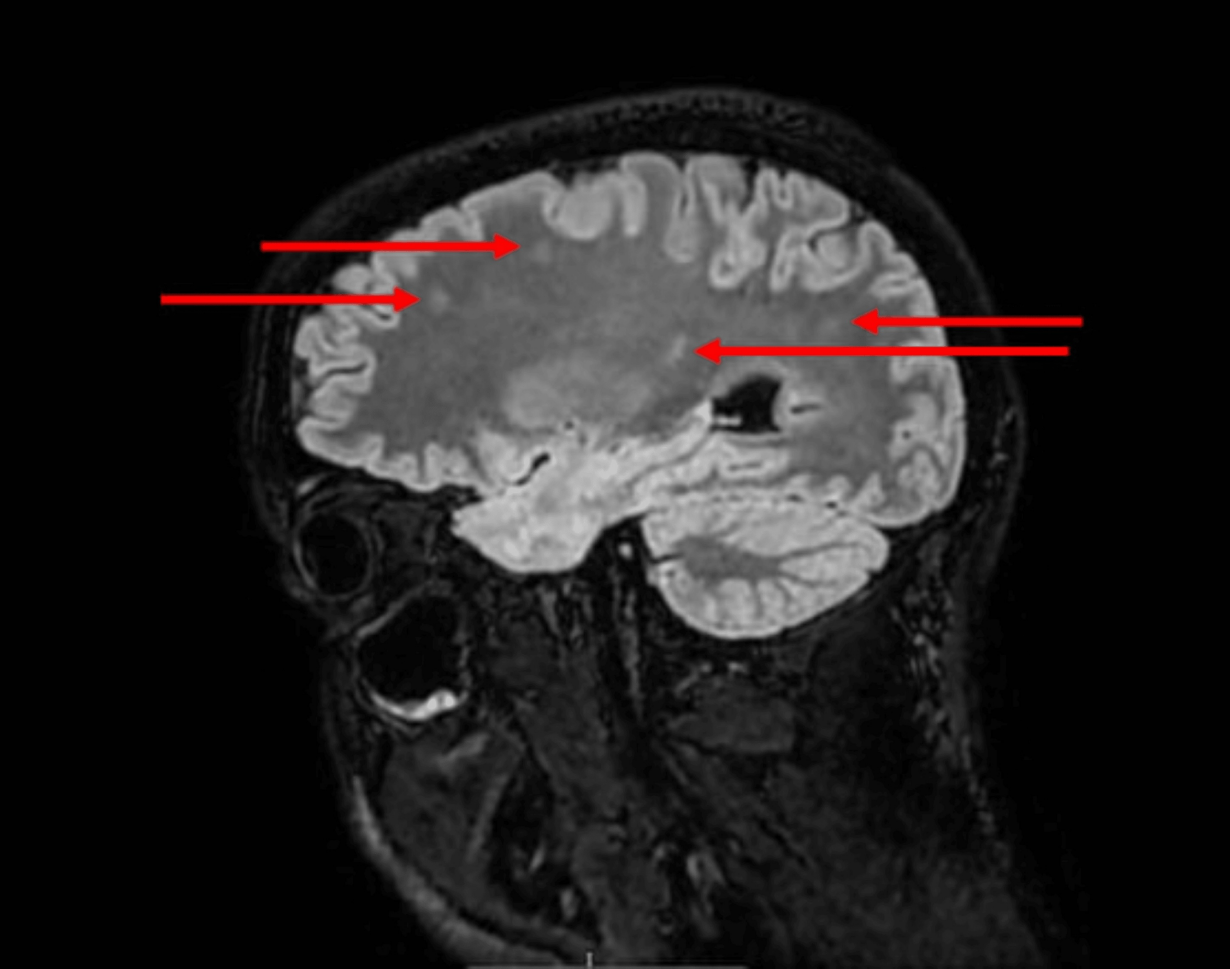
Brain MRI Brain MRI showing multifocal white matter lesions (red arrows).
